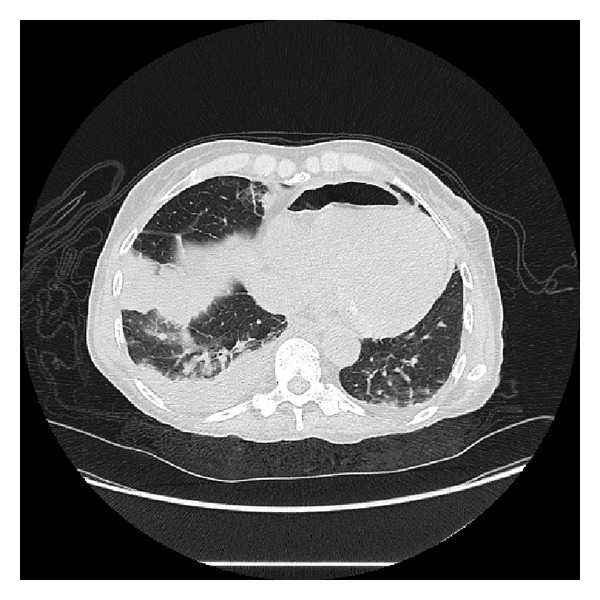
Thoracic CT reveals a loop of bowel incarcerated into the pericardial cavity. This image can be easily mistaken for pneumopericardium secondary to postoperative changes. Right lower lobe consolidation may be appreciated as well.
